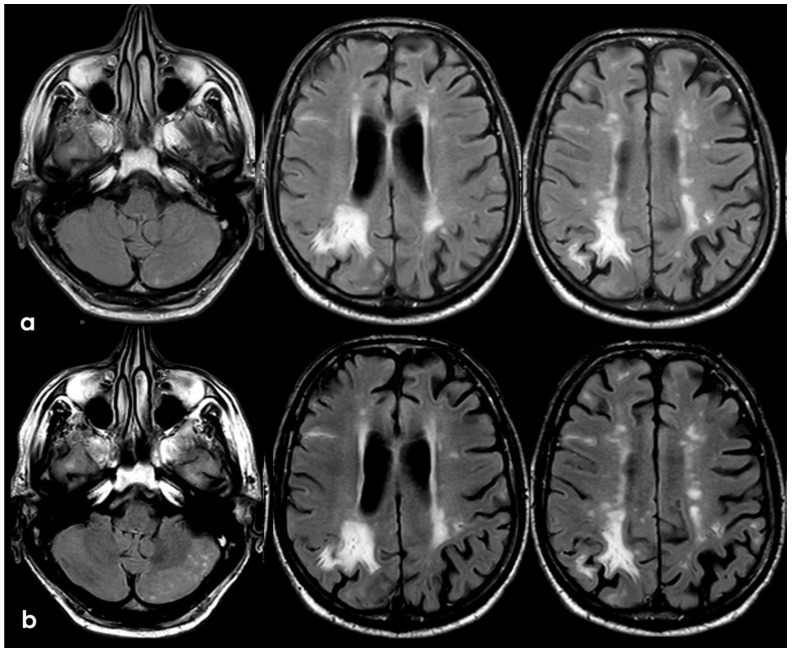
Brain MRI (axial FLAIR) before and after contrast administration (panel (a) and (b), respectively), showing a spontaneous hyperintense signal on several sulci (panel (a)) in the left cerebellar lobe (left column) and in the right frontal lobe (middle and right columns), all with mild enhancement after contrast administration (panel (b)) and cortical ribbon swelling in the corresponding giri.
